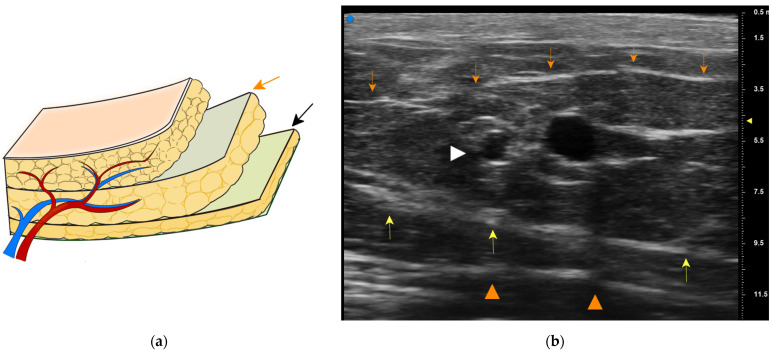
(a) Showing two distinct planes: the superficial fascia (orange arrow) and the Camper fascia (black arrow). (b) High-resolution ultrasonographic imaging of the SCIA superficial branch. The superficial branch (white arrow head) is identified between the superficial fascia (orange arrows) and the Camper fascia (yellow arrows). The Scarpa fascia is also distinct (orange arrow heads).
